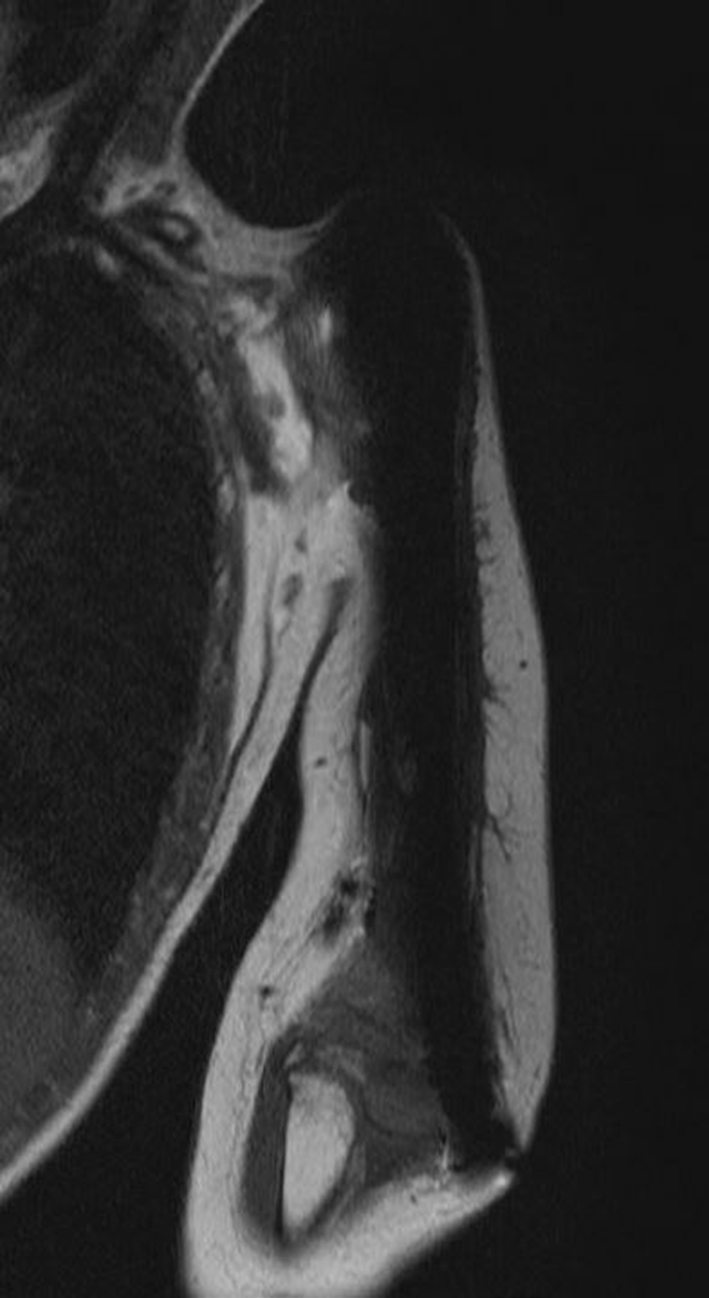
Coronar MRI image of a dislocated spacer with distal dislocation after total humerus resection and scarce soft tissue coverage (patient #4)
